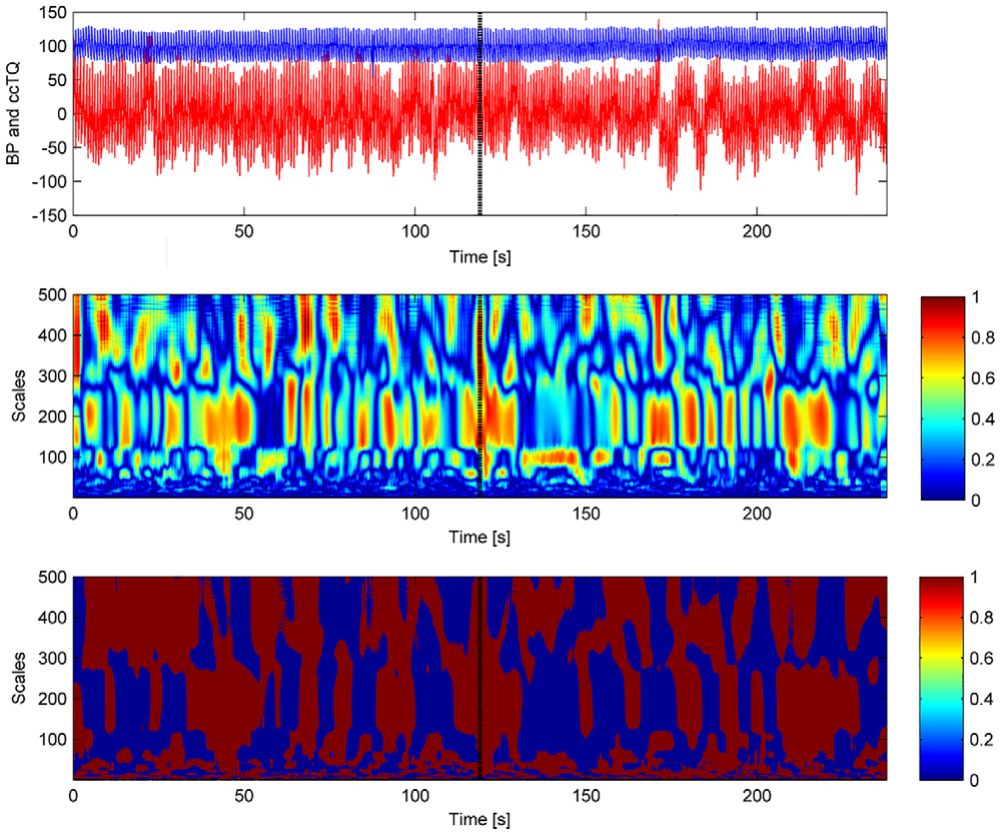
Representative WCO (middle panel) and WPCO (lower panel) tracings. BP (red) and cc-TQ (blue) signals are provided in the upper panel. WCO and WPCO remains relatively stable throughout baseline and CT. Peak values at ~1 Hz and ~0.3 Hz are visible. The black marker indicates the start of CT.
